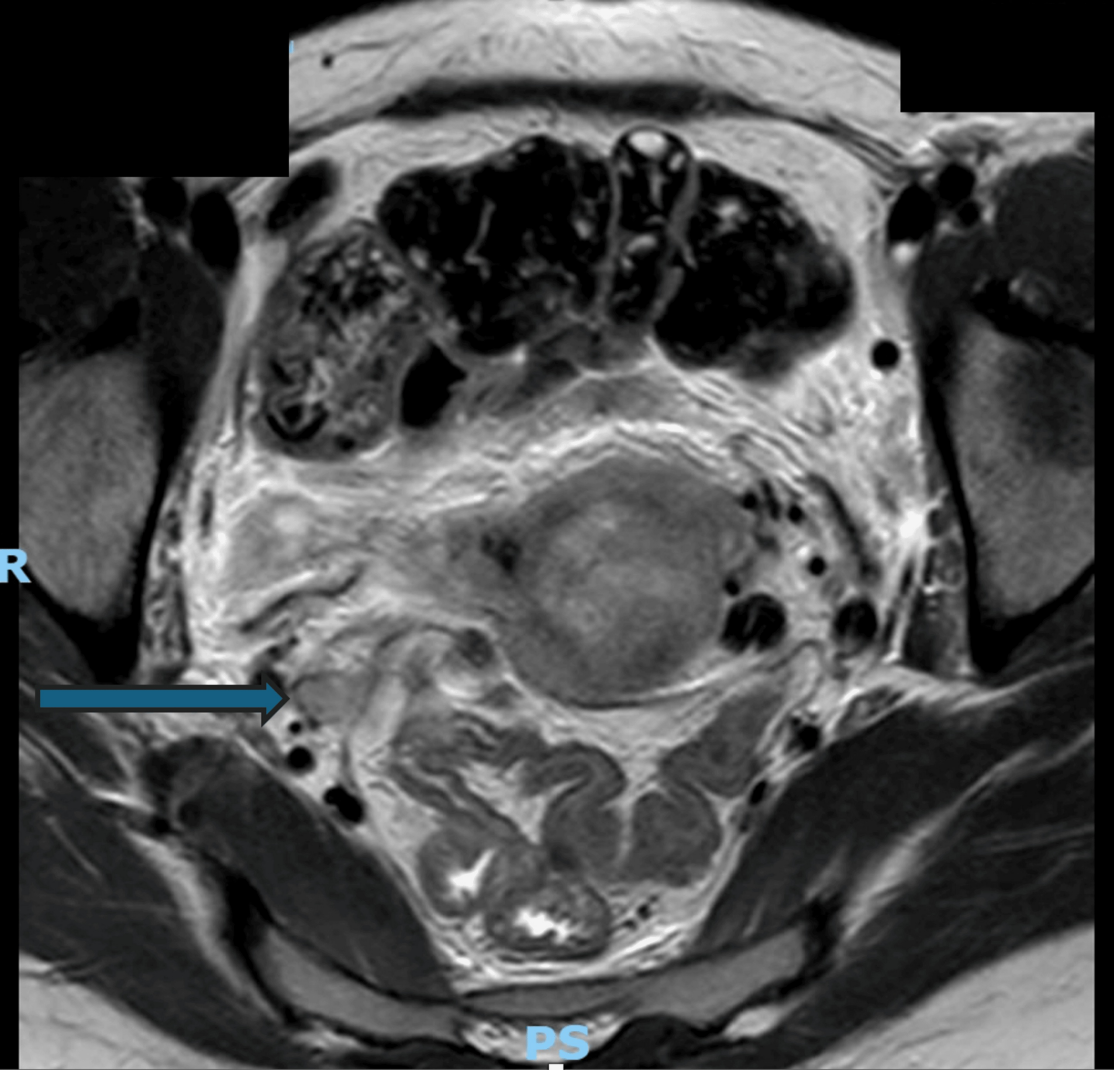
Axial T2-weighted pelvic MRI showing lymph node involvement. Axial T2-weighted MRI demonstrates an irregular, heterogeneously hyperintense cervical mass. The blue arrow indicates enlargement of the right internal iliac lymph node.
